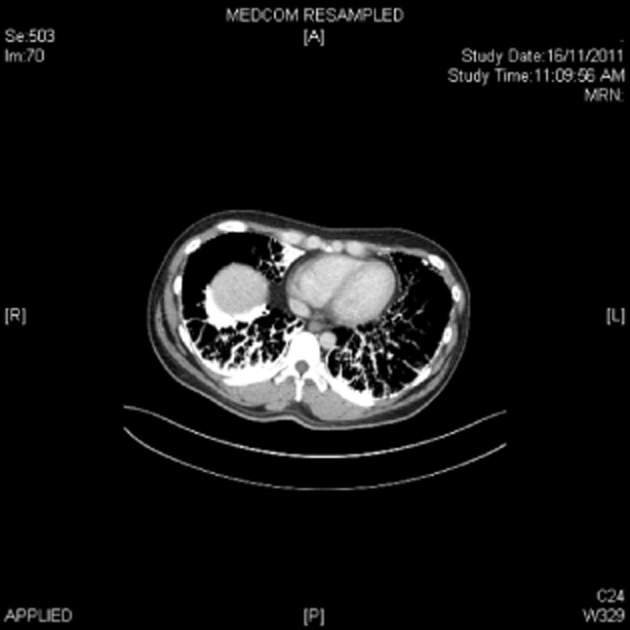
Computed tomograph in the bone window showing typical calcifications due to accumulations of microliths in the alveoli.
